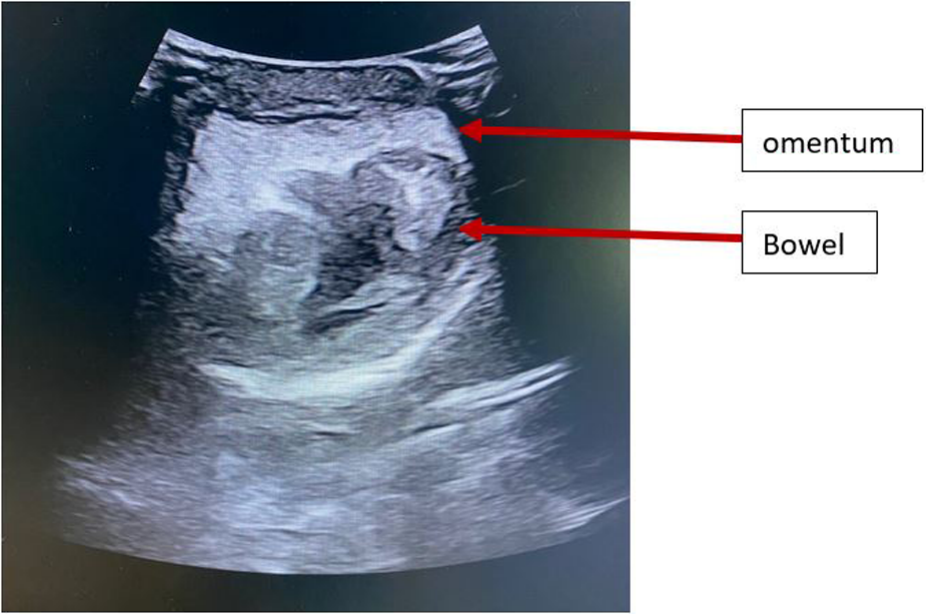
Ultrasonographic image of the vaginal prolapse showing the displaced omentum and bowel loops.
